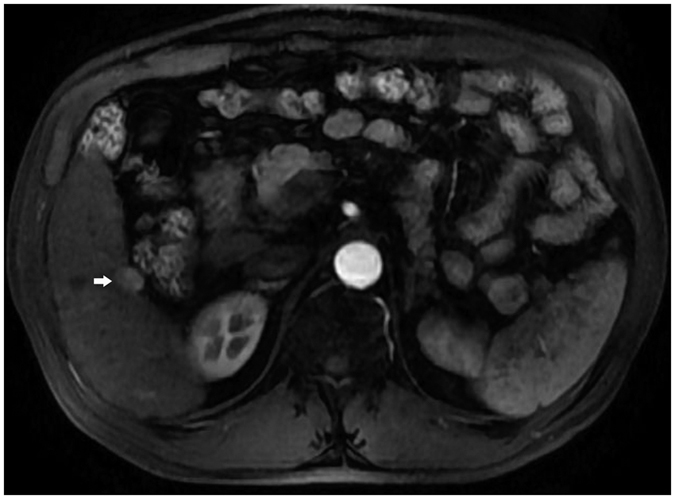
Lesion (white arrow) near the gastrointestinal tract as shown with enhanced MRI.
